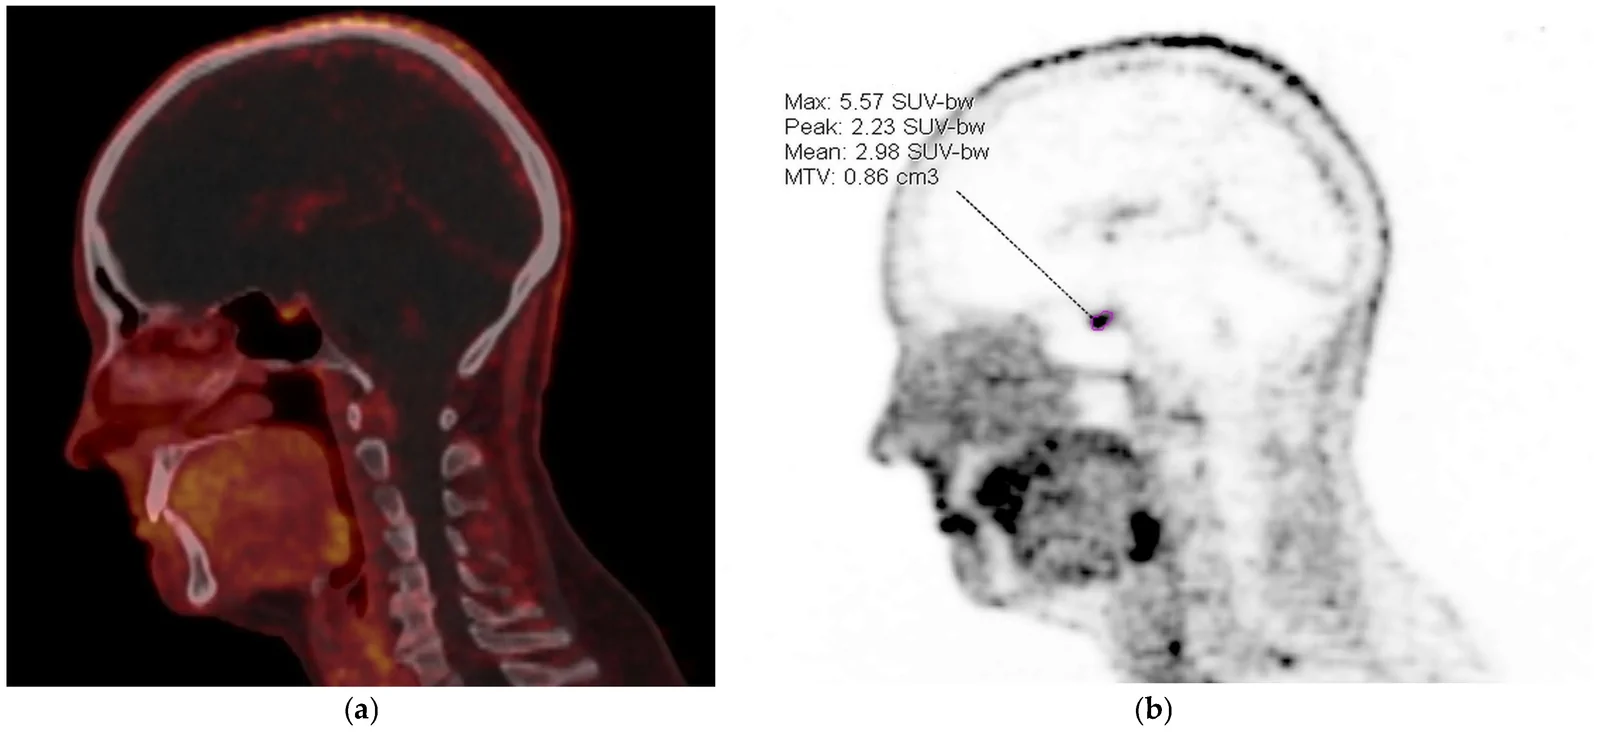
Representative PET/CT images were obtained from a 68-year-old male patient with pancreatic ductal carcinoma. Written informed consent for publication of anonymized images was obtained. (a) Sagittal ^68^Ga-Trivehexin(R) PET of neurocranium with marked pituitary tracer uptake. (b) Sagittal fused ^68^Ga-Trivehexin (R) PET/CT with marked tracer uptake of the pituitary gland in the sella turcica. The metabolically active lesion is delineated by a volume of interest (VOI) isocontour, with a maximum standardized uptake value normalized to body weight (SUV-bw) of 5.57, peak SUV-bw of 2.23, mean SUV-bw of 2.98, and a metabolic tumor volume (MTV) of 0.86 cm^3^.
